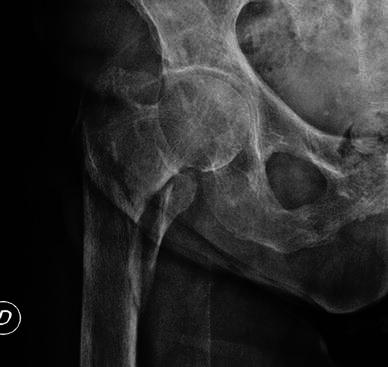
Preoperative anteroposterior radiograph shows an AO-OTA 31-A2.2 hip fracture
